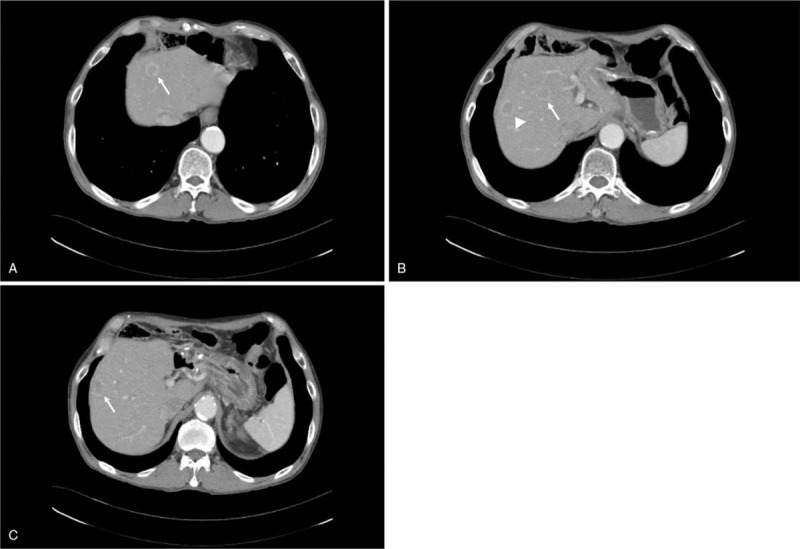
Abdominal computed tomography showed mutiple targetoid masses in both lobe of the liver (arrow). The largest 1 is 2.4 cm in S6 subcapsular area (arrow head).
